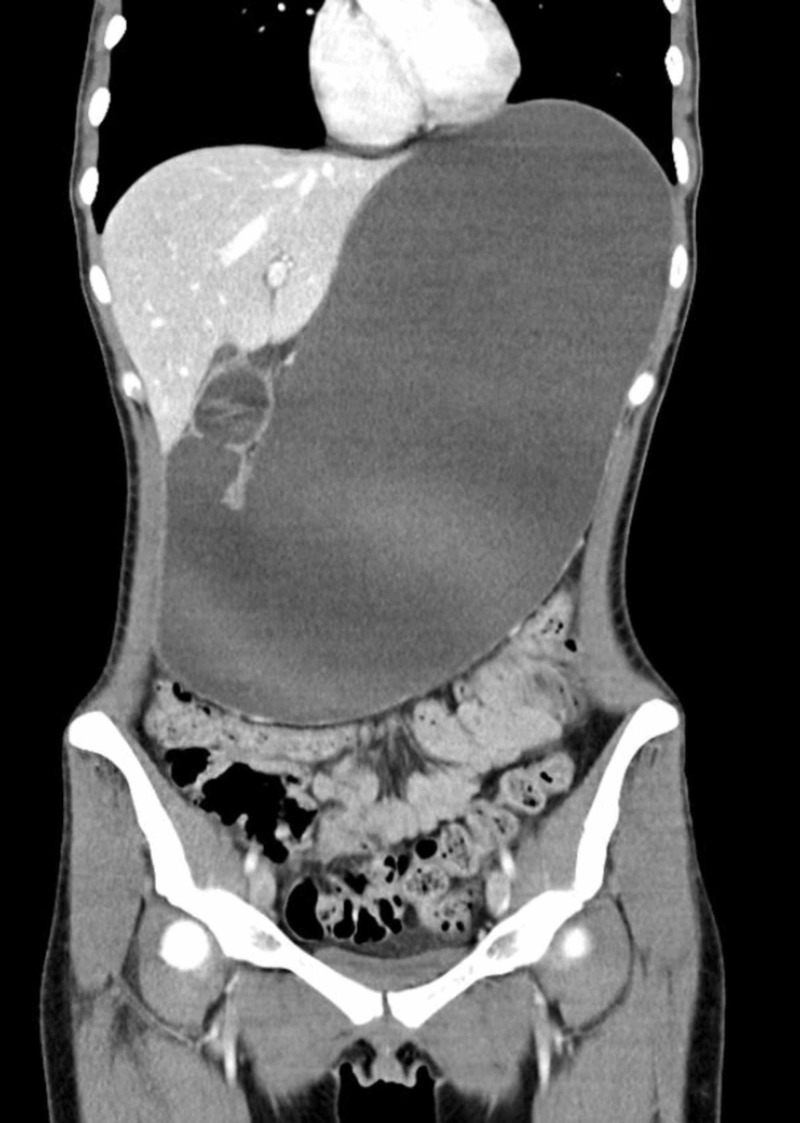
IV contrast-enhanced coronal computed tomography (CT) of the abdomen and pelvis consistent with small bowel obstruction. This figure demonstrates severe dilatation of the stomach and proximal duodenum consistent with small bowel obstruction. Portions of the distal small and large bowel are decompressed.
